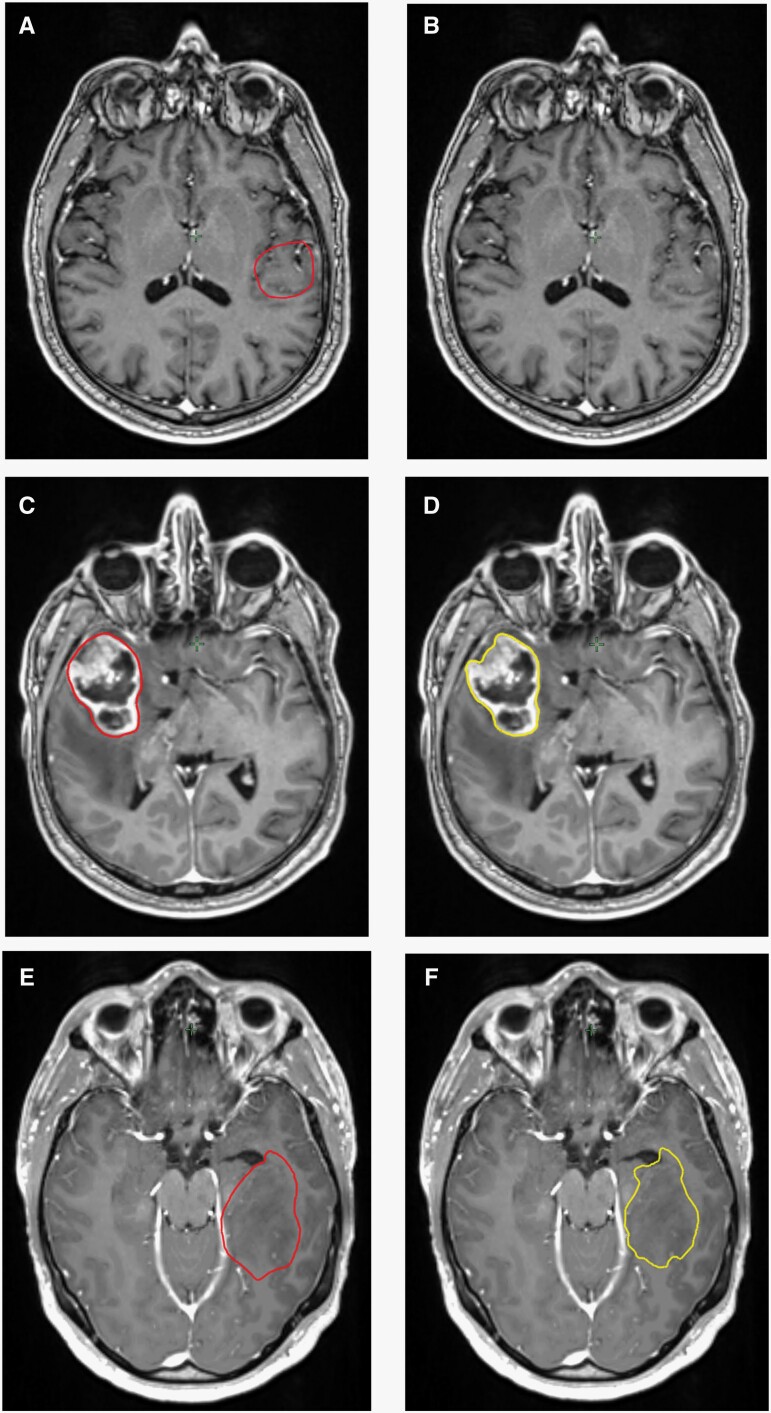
HD-GLIO predictions on clinical radiation therapy-patients. Red segment = manual gross target volume (GTV), Yellow segment = HD-GLIO prediction. (A) Manual GTV on patient holding atypical GBM expression, where (B) HD-GLIO failed to predict tumor volume. (C) Manual vs. (B) score-4 HD-GLIO prediction. (E) Manual GTV vs. (F) score-3 HD-GLIO prediction. All images: C-T1-w MRI.
